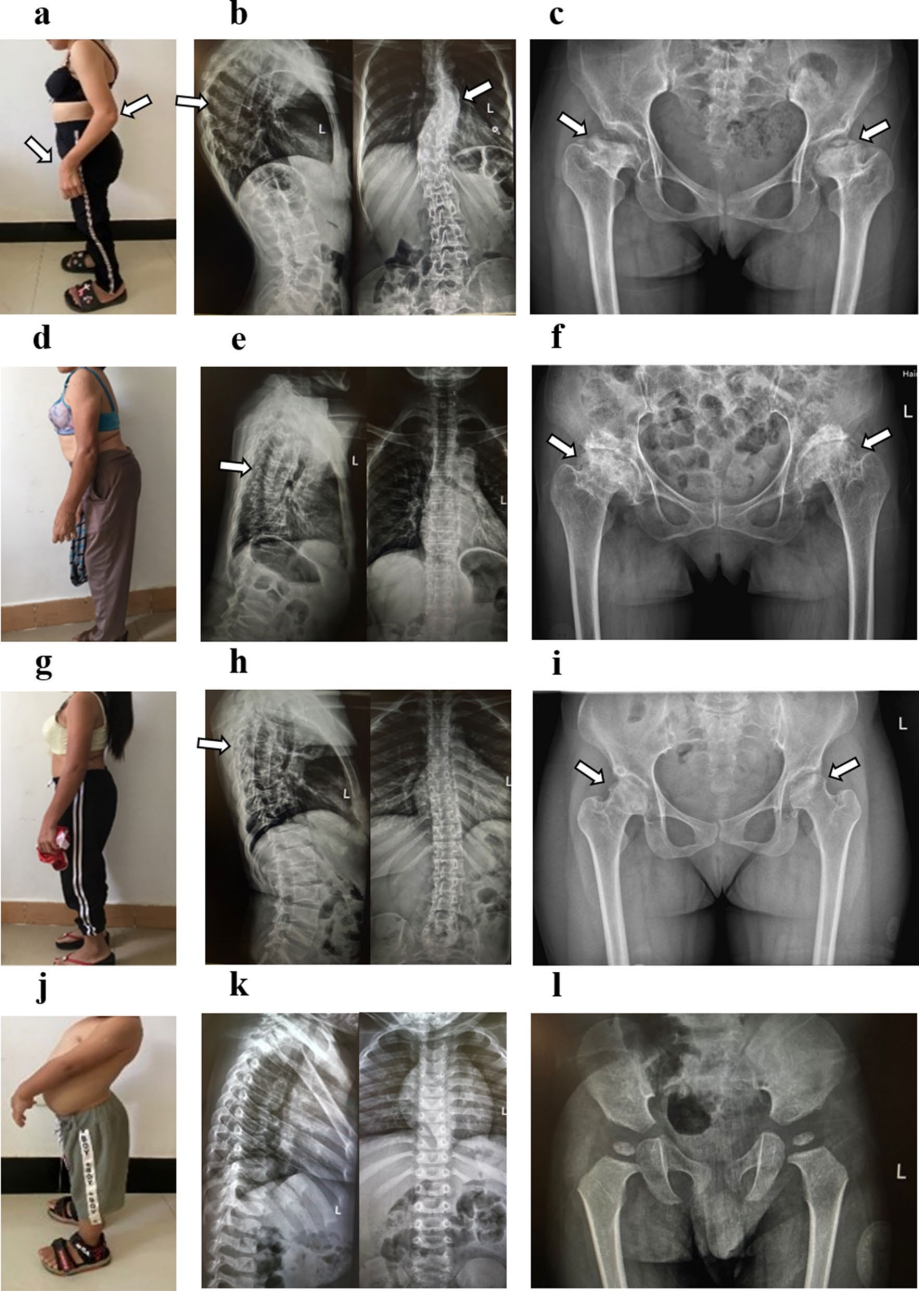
General appearance photographs and skeletal system radiographs of patients (II3: a–c, I2: d–f, II2: g–i, III1: j–l) in Family 1,908,322. All the patients presented with disproportionate short neck and trunk and had no deformities in fingers (a, d, g, j). The proband (II3) presented with barrel chest, deformity of elbow and wrist joints (a). The youngest patient (III1) presented with pigeon chest and had normal fingers (j). The spine X-ray photographs of adult patients revealed kyphoscoliosis (b) or kyphosis (e, h). The hip joint X-ray showed bilateral femoral heads ischemia or aseptic inflammation, bilateral hip joints subluxation, bilateral acetabular fossa shallowing, bilateral femoral heads collapsing, bone destruction on articular surface, irregular and low-density shadows under the articular surface, unclear boundary, narrowing of joint space, and shortening of femoral neck (c, f, l). The radiographs of III1 were normal (k–l)
